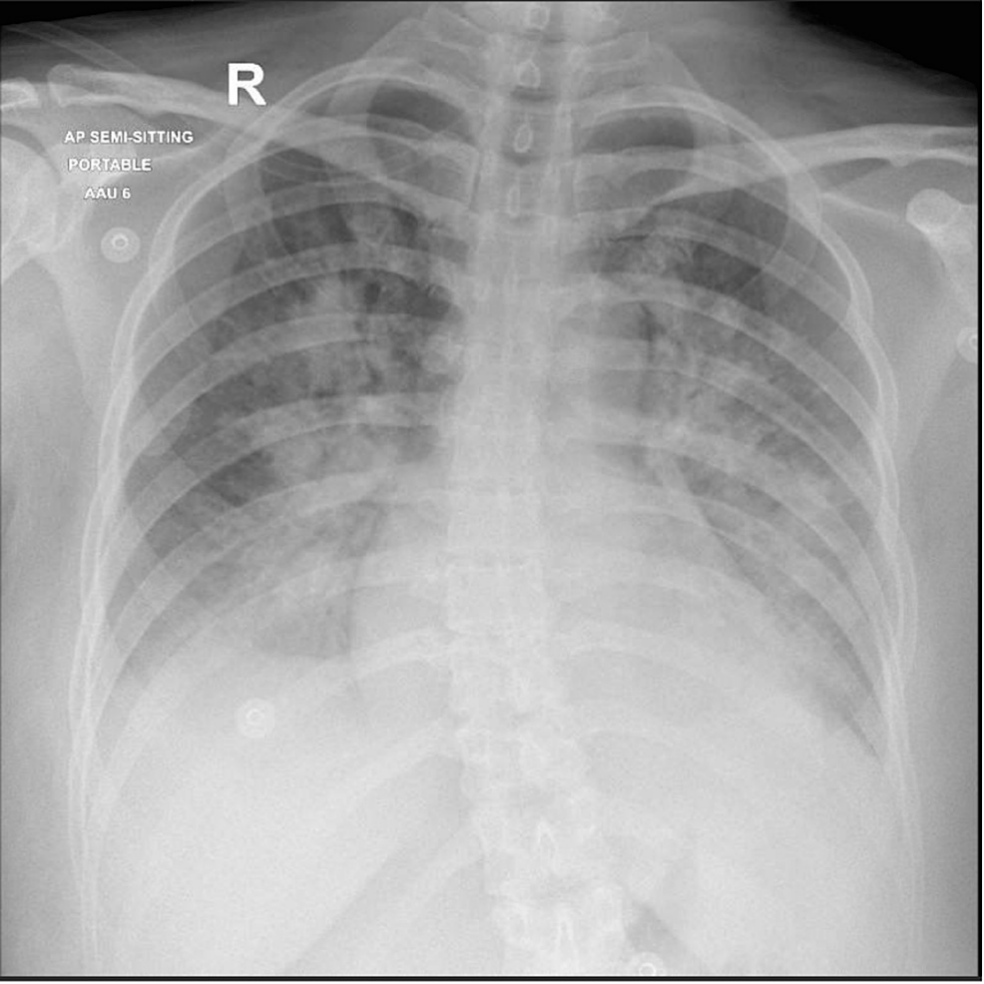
Repeat chest X-ray showing features of diffuse pulmonary edema
